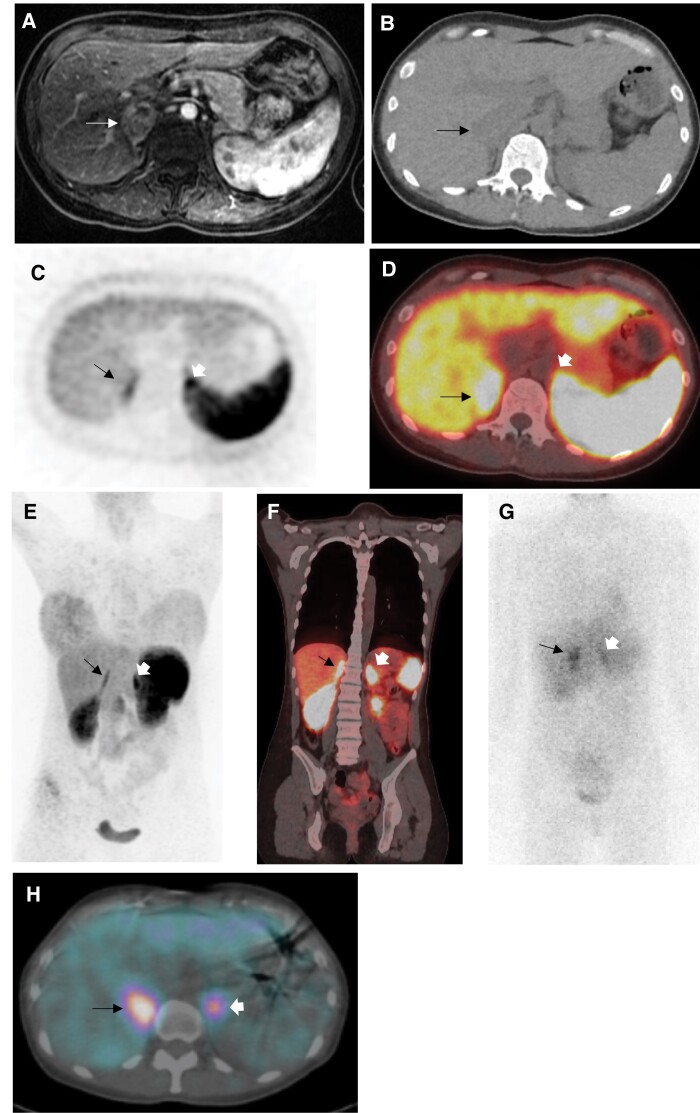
T1-weighted postcontrast axial magnetic resonance imaging (MRI) showing a 2.7 × 2.7 × 4.1-cm enhancing mass in the right adrenal gland, with punctate hyperintensity in the periphery of the lesion likely reflecting calcifications or blood products (A). ^68^Ga-DOTATATE PET/CT failed to demonstrate avidity within the right adrenal mass. A CT image from the ^68^Ga-DOTATATE PET/CT study shows the right adrenal mass (B). Axial standalone PET and fused ^68^Ga-DOTATATE PET/CT images were interpreted as showing physiologic uptake in both adrenal glands (right adrenal = arrow, left adrenal = arrowhead) (C and D). The ^68^Ga-DOTATATE PET maximum intensity projection (MIP) whole-body image demonstrated similar findings when compared with axial (E). Measurement of SUVmax on the fused coronal ^68^Ga-DOTATATE PET/CT shows that the right adrenal gland (SUVmax 9.7) had less DOTATATE uptake than the left adrenal gland (SUVmax 15.5) (F). ^123^I-MIBG imaging showed significantly increased uptake in the right adrenal mass consistent with pheochromocytoma. The right adrenal gland had greater ^123^I-MIBG uptake than the left adrenal gland on the anterior whole body planar image (G). The fused axial ^123^I-MIBG SPECT/CT shows focally and asymmetrically intense uptake in the right adrenal mass compatible with pheochromocytoma (H). The relatively high ^123^I-MIBG uptake in the left adrenal gland was interpreted as physiologic.
